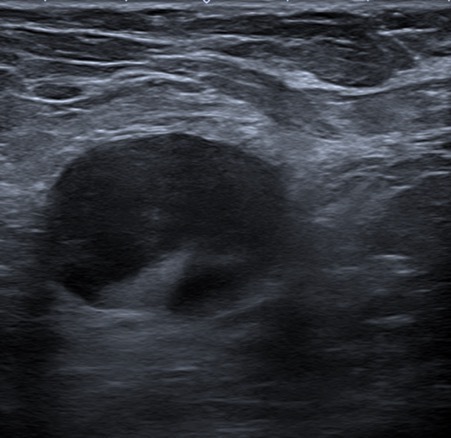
Ultrasound of the left breast with enlarged axillary lymph nodes.
